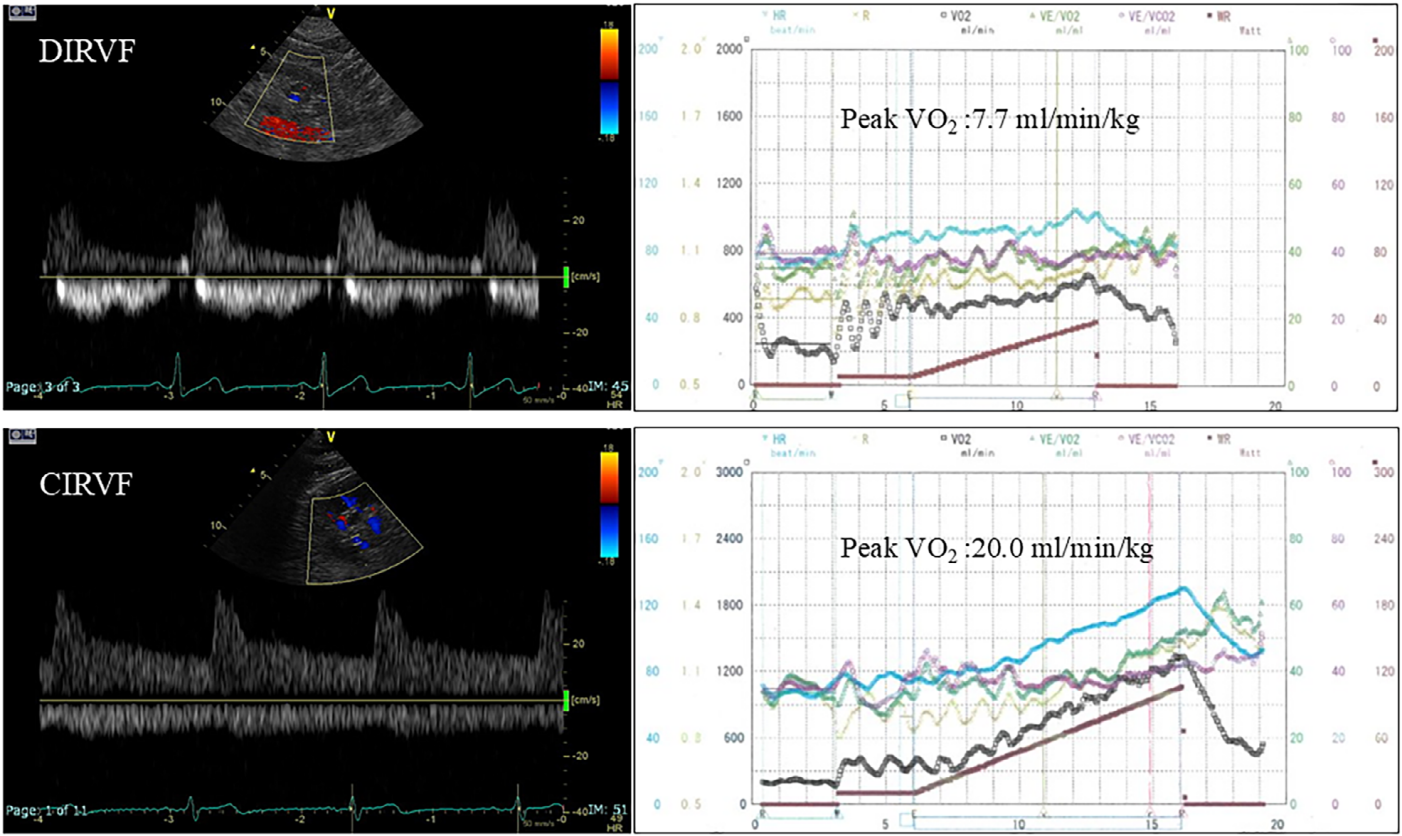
(Left) Continuous (lower row) and discontinuous (upper row) Doppler‐derived intrarenal venous flow. (Right) Corresponding findings of cardiopulmonary exercise testing. CIRVF, continuous Doppler‐derived intrarenal venous flow; DIRVF, discontinuous Doppler‐derived intrarenal venous flow.
